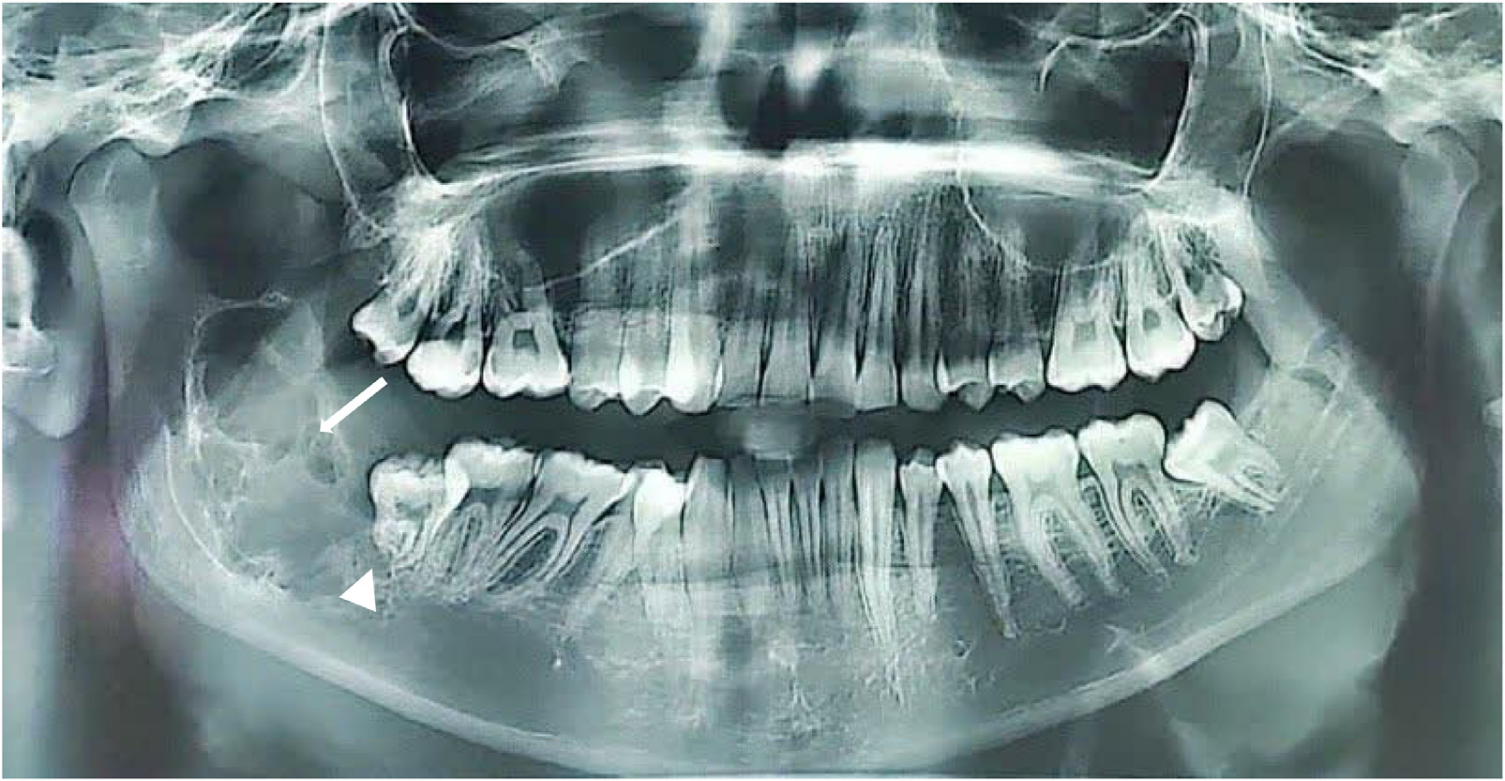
Panoramic radiograph (orthopantomogram, OPG) in a 2D projection plane demonstrating a multilocular radiolucent lesion in the posterior mandible and ascending ramus associated with a semi-erupted right mandibular third molar (#48), which is vertically positioned, inferiorly and lingually oriented. The lesion features curved and coarse septa in addition to typical wispy septa (white arrows), irregular bone destruction with a hazy and blurred pattern, and possible root resorption of tooth #48 (white arrowhead). A normally erupted contralateral third molar (#38) is visible for comparison.
